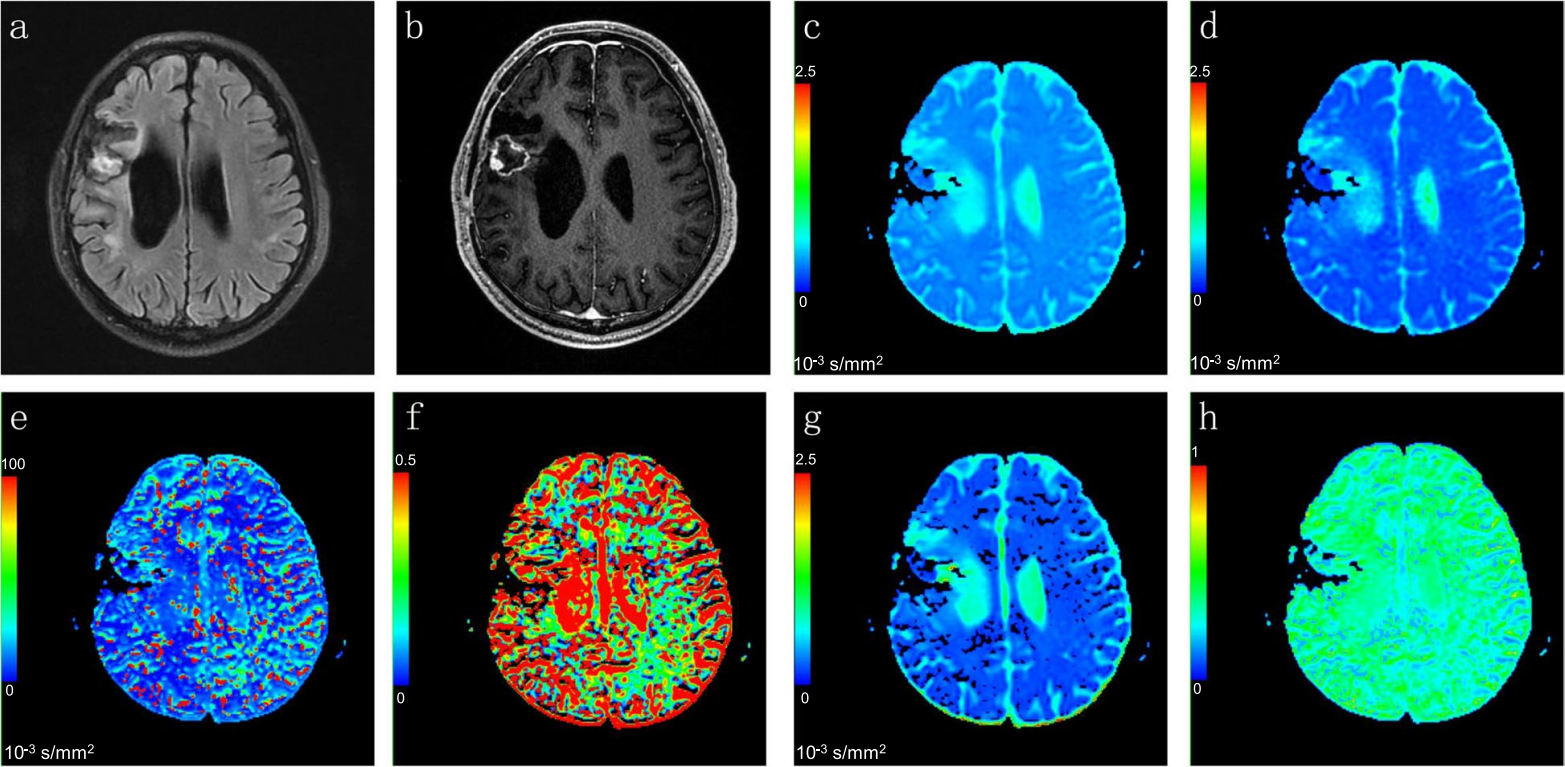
Representative images of a patient with pseudoprogression. Axial FLAIR (a) and contrast-enhanced T1WI (b) showed a necrotic contrast-enhancing lesion in the right temporal lobe. The lesion disappeared after 6 cycles of temozolomide chemoradiation, which was defined as pseudoprogression. The ADC (c), D (d), D* (e), f (f), DDC (g) and α (h) maps were calculated automatically from the MADC software
